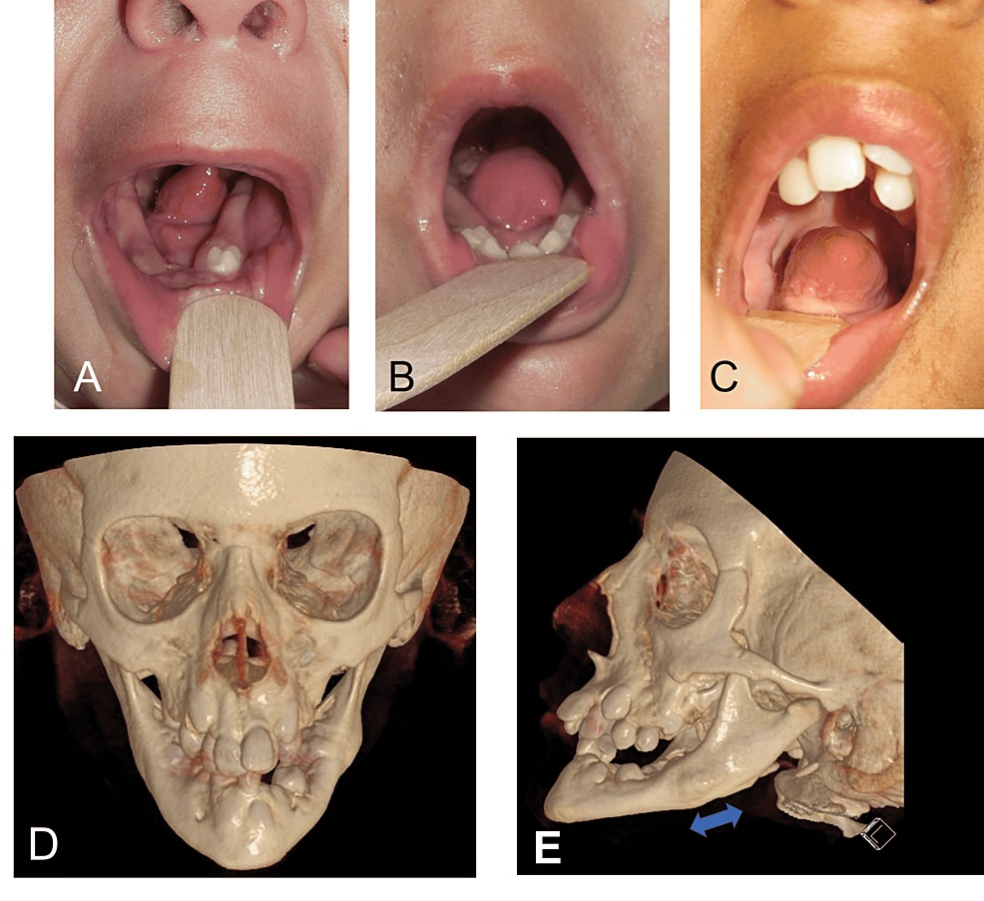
Case 2 (A) Microglossia of the anterior tongue with glossoptosis, at 16 months of age. (B) Correction of glossoptosis following mandibular distraction osteogenesis, at 18 months of age. (C) Sustained correction of oral tongue positioning, at 10 years of age. (D) 3D maxillofacial CT, at age 10, nine years after bilateral mandibular distraction osteogenesis using external multivector distractors. The left panel shows severe mandibular and maxillary constriction with telescopic bite. (E) The blue arrow indicates the region of regenerated bone.
